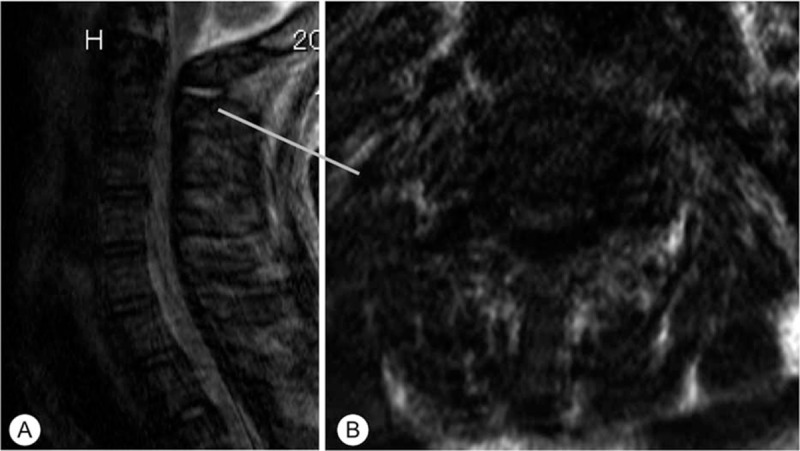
Preoperative magnetic resonance imaging. Sagittal T2-weighted images showing cervical spinal cord compression from C1 to C4 (A). T1-weighted imaging showed that the spinal cord was most compressed at the C1/2 level (B).
